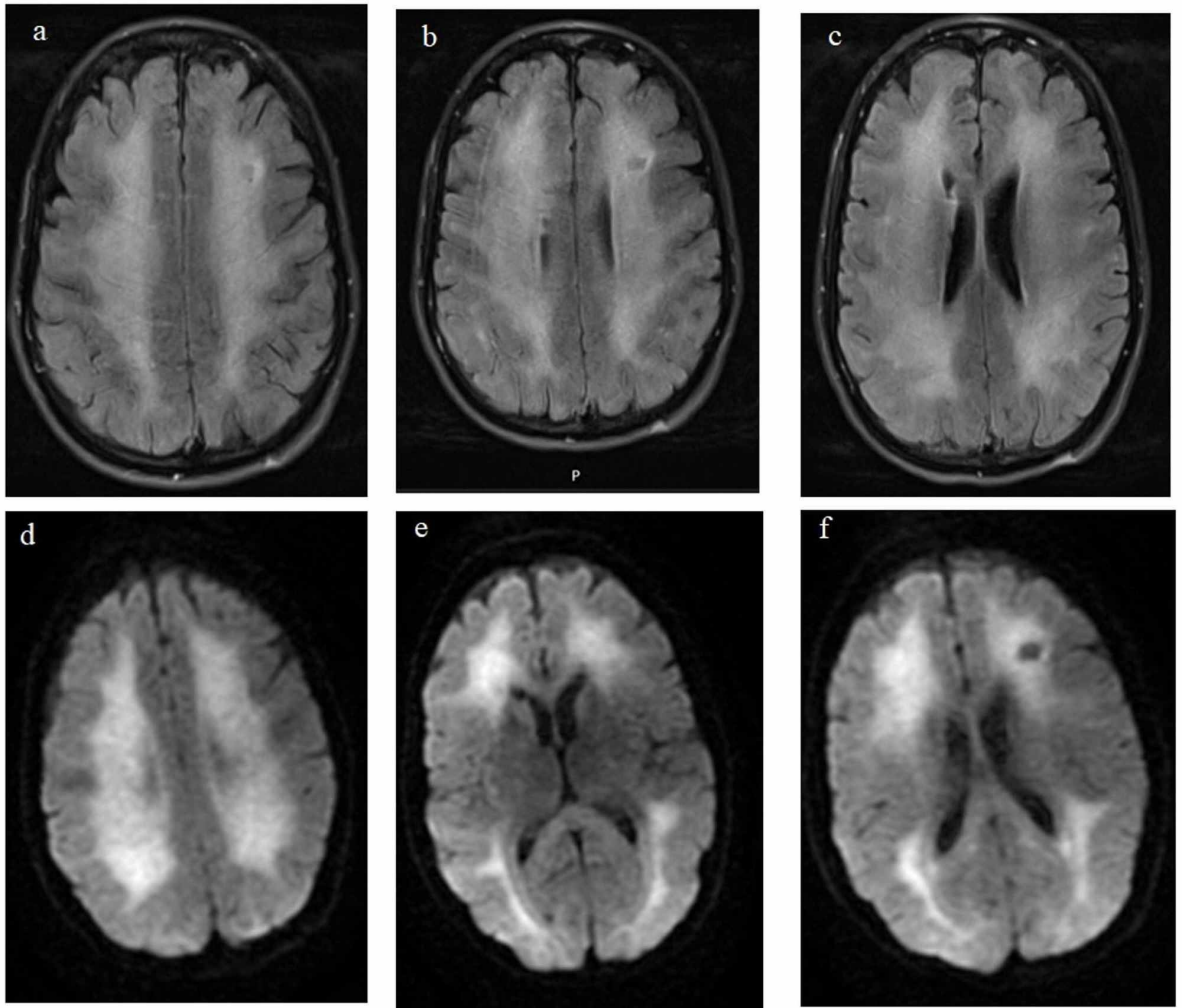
MRI images of the brain showing diffuse cortical and subcortical white matter FLAIR hyperintensity and diffusion restriction. A-C: FLAIR sequence. D-F: DWI sequence. DWI - diffusion-weighted imaging; FLAIR - fluid-attenuated inversion recovery
